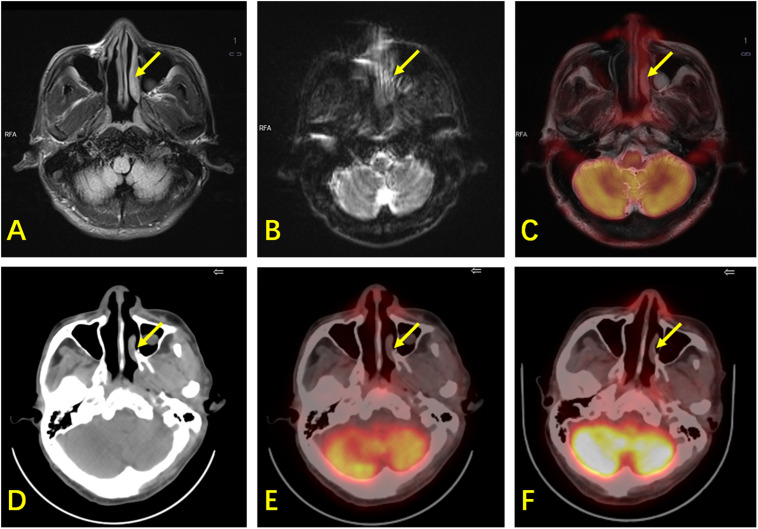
A 61-year-old man with ENKTL. (A) T2WI shows mild thickening of the nasopharynx and left nasal cavity mucosa (arrow) with hyperintensity. (B) DWI shows limited diffusion of the lesion. (C) PET/MR shows mild ^18^F-FDG uptake (SUVmax is 2.6, while the SUVmax of the reference liver is 2.3) and hyperintensity of T2WI of the lesion with suspected malignancy. (D) CT shows mild thickening of the nasopharynx and left nasal cavity mucosa. (E)
